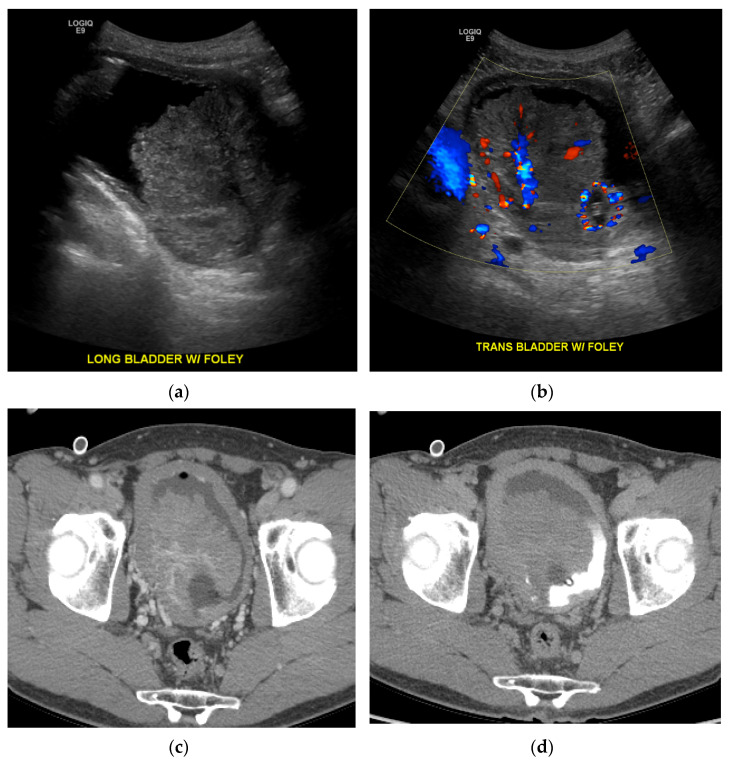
Ultrasound and CT images of non-muscle invasive bladder cancer. (a) Longitudinal grayscale ultrasound image of the bladder shows a large mass within the bladder. (b) Transverse color Doppler image shows vascularity within the mass. (c) Axial portal venous phase CT image shows a central area of enhancement, similar to the color Doppler ultrasound image. (d) Axial delayed phase CT image shows the mass partially surrounded by excreted contrast in the bladder.
